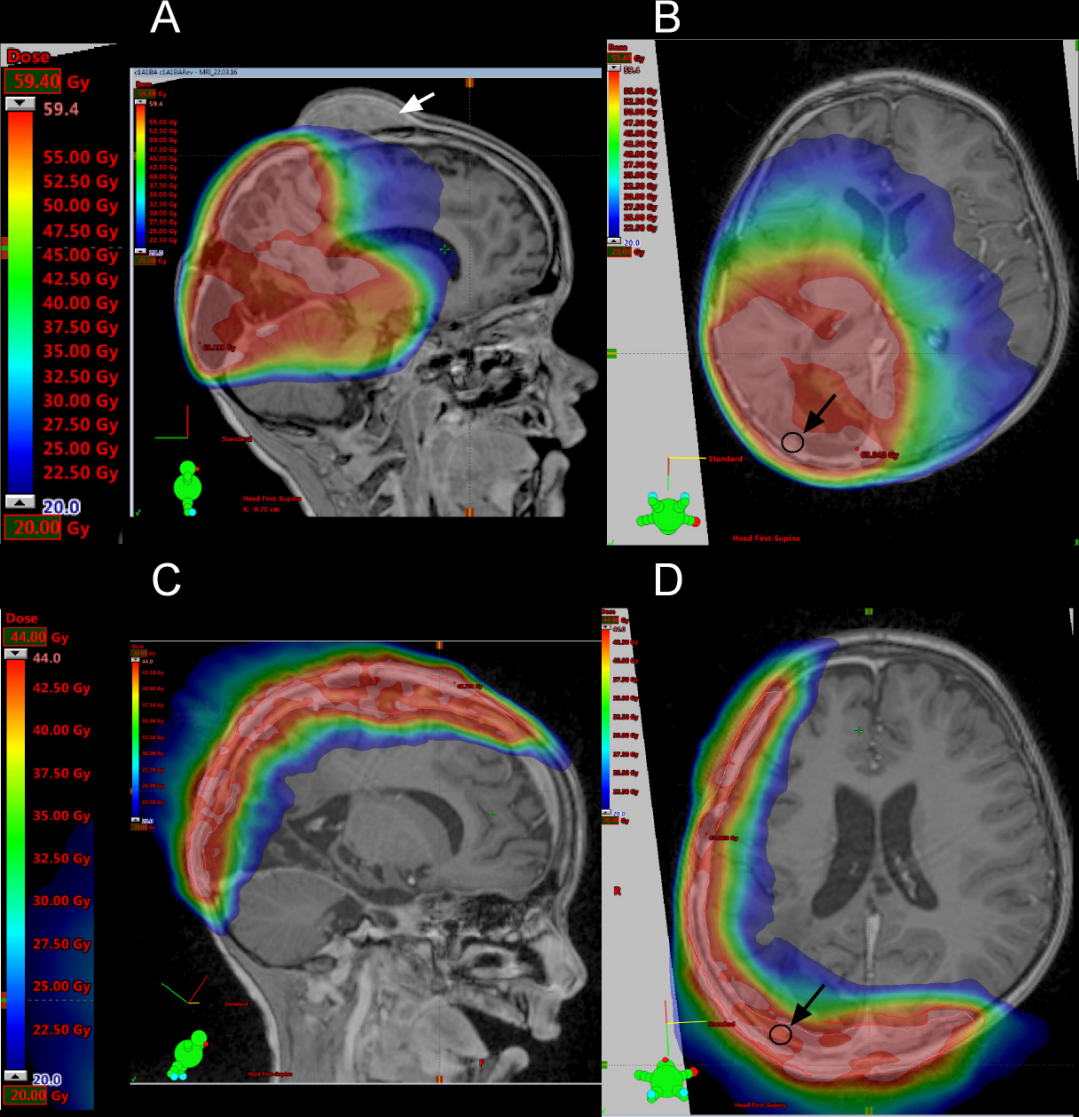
Irradiation fields used in the treatment protocol (A-B) MRI showing the irradiation field used in the first line therapy. The intensity of the irradiation field is shown in color. The gradation is from 20 Gy (blue) to 59.4 Gy (red). (C-D) MRI showing the irradiation field used in the targeted therapy. The gradation is from 20 Gy (blue) to 44 Gy (red). The white arrow indicates a skull metastasis, the dark circle and the dark arrow indicate a dural lesion.
